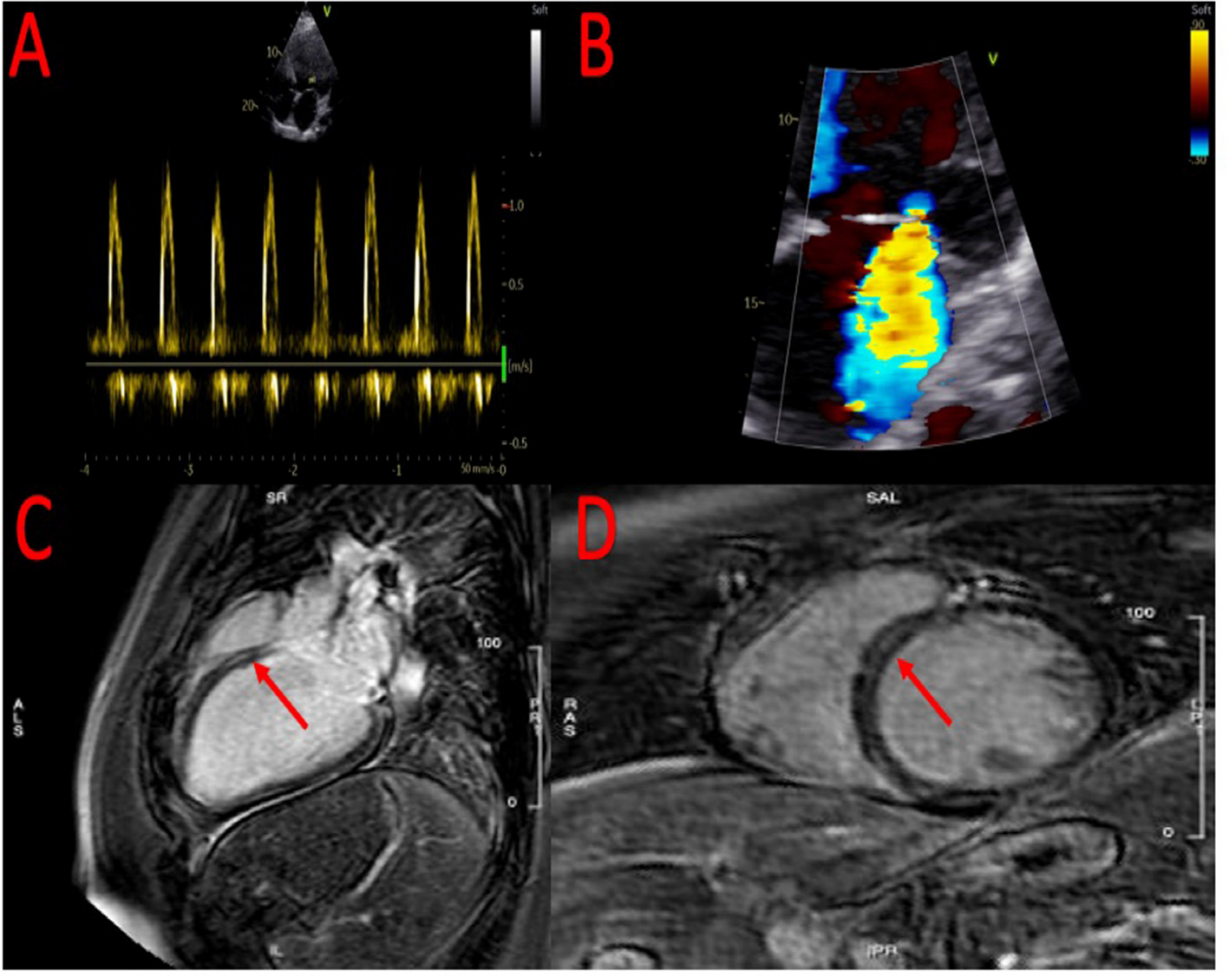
Echocardiographic evidence of severe diastolic dysfunction (A) and severe mitral regurgitation (B). At cardiac magnetic resonance, evidence of small area of fibrosis at the basal level of the interventricular septum (C,D).
